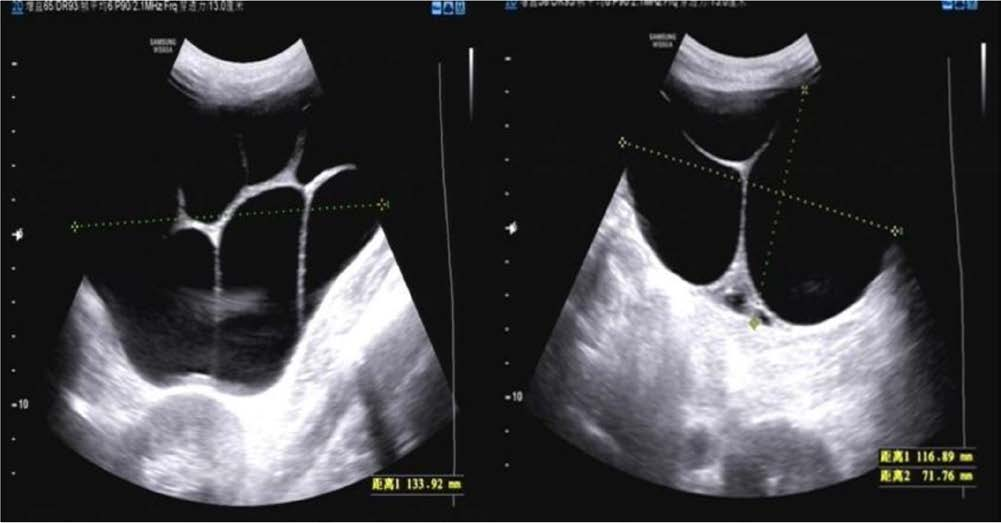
A 21-yr-old primigravida at 9 2/7 wk of gestation, presented with a 7-d history of abdominal distension. Ultrasonographic imaging revealed bilateral ovarian enlargement with multiple contiguous thin-walled cysts containing anechoic fluid.
